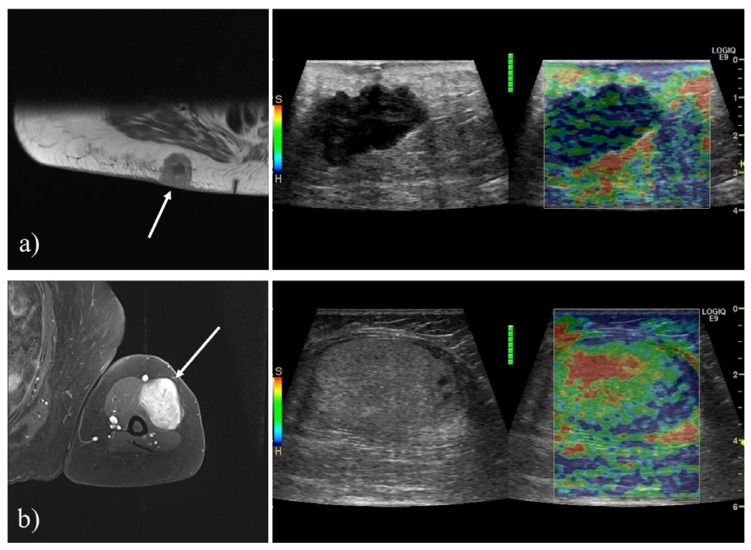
Examples of tumors included. (a) A 71-year-old female with metastasis of malignant melanoma. T1-weighted transverse contrast-enhanced MRI showing a lesion superficial to the right gluteal musculature. The tumor appeared hypoechoic and irregular on B-mode US, and SE revealed the tumor to be hard (blue). (b) A 75- year-old female with a chondroid lipoma. T1-weighted fat suppressed contrast enhanced MRI showing a tumor in the anterolateral upper arm. B-mode US and strain elastography (SE) revealed the tumor to be isoechoic, homogenous and soft (red).
